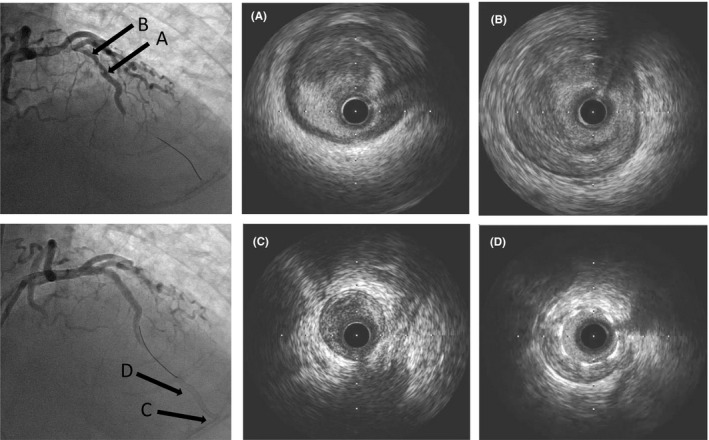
After thrombus aspiration, no translucencies were observed in the lesion or in distal blood flow (upper row). Initial intravascular ultrasonography (IVUS) showed a low‐density area suspected of representing thrombi in the 12 o'clock direction (A) and a large amount of plaque in the proximal site (B). Final angiography showed no distal blood flow (lower row). Final IVUS showed thrombi and plaque at the distal end of the stent (C) and no intrastent blood flow (D).
